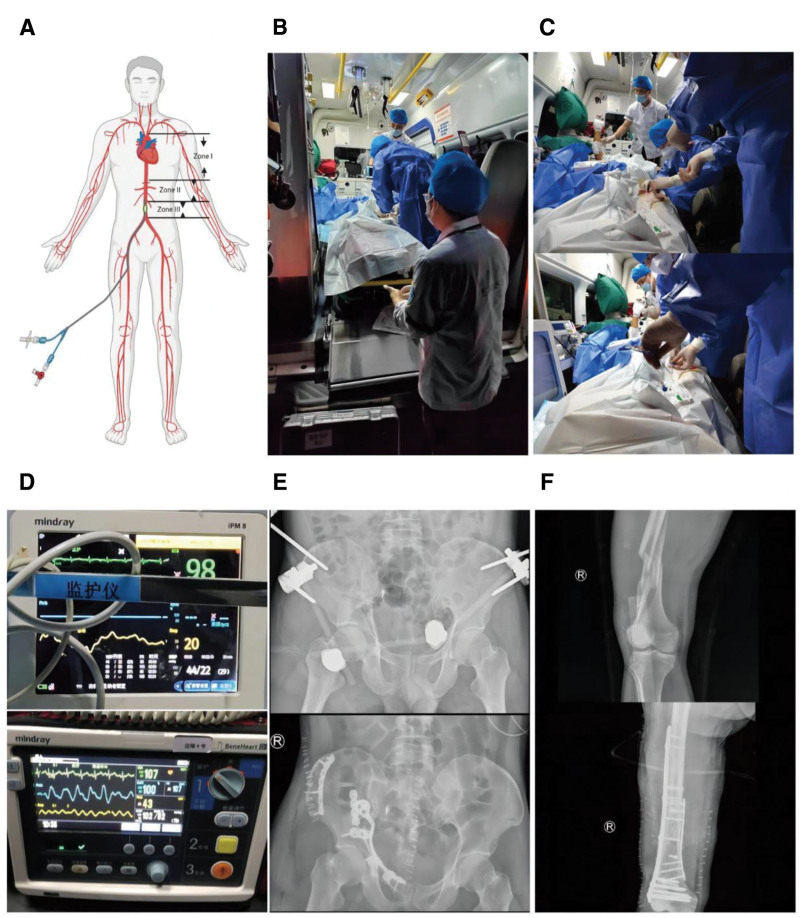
Inhospital and prehospital treatment process. (A) Aortic zones in REBOA conceptualization, (B, C) REBOA deployment by trauma resuscitation team in ambulance, (D) vital sign comparison: pre- versus post-REBOA, (E, F) preoperative and postoperative radiographs of pelvic and femoral internal fixation. REBOA = resuscitative endovascular balloon occlusion of the aorta.
